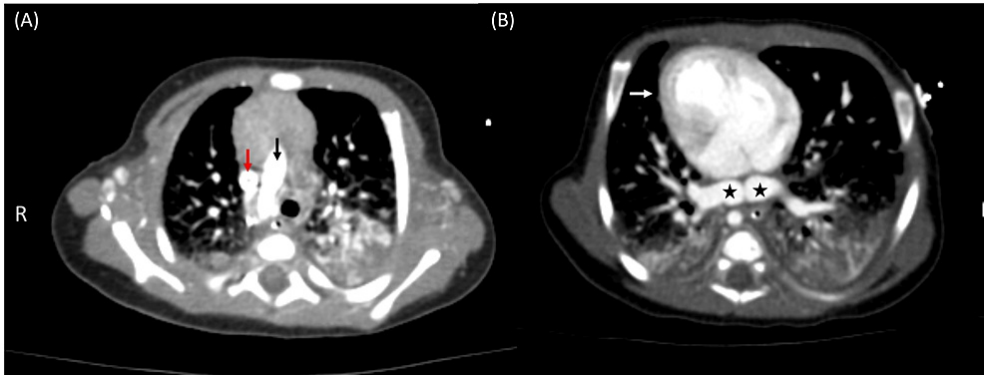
(A) Axial CT scan at the upper part of the chest shows a right-sided aortic arch (black arrow) and a normal right SVC (red arrow). (B) Axial CT scan at the level of the chest: a dextrocardia (white arrow) with abnormally dilated bilateral inferior pulmonary veins (stars). CT: Computed tomography; SVC: Superior vena cava
